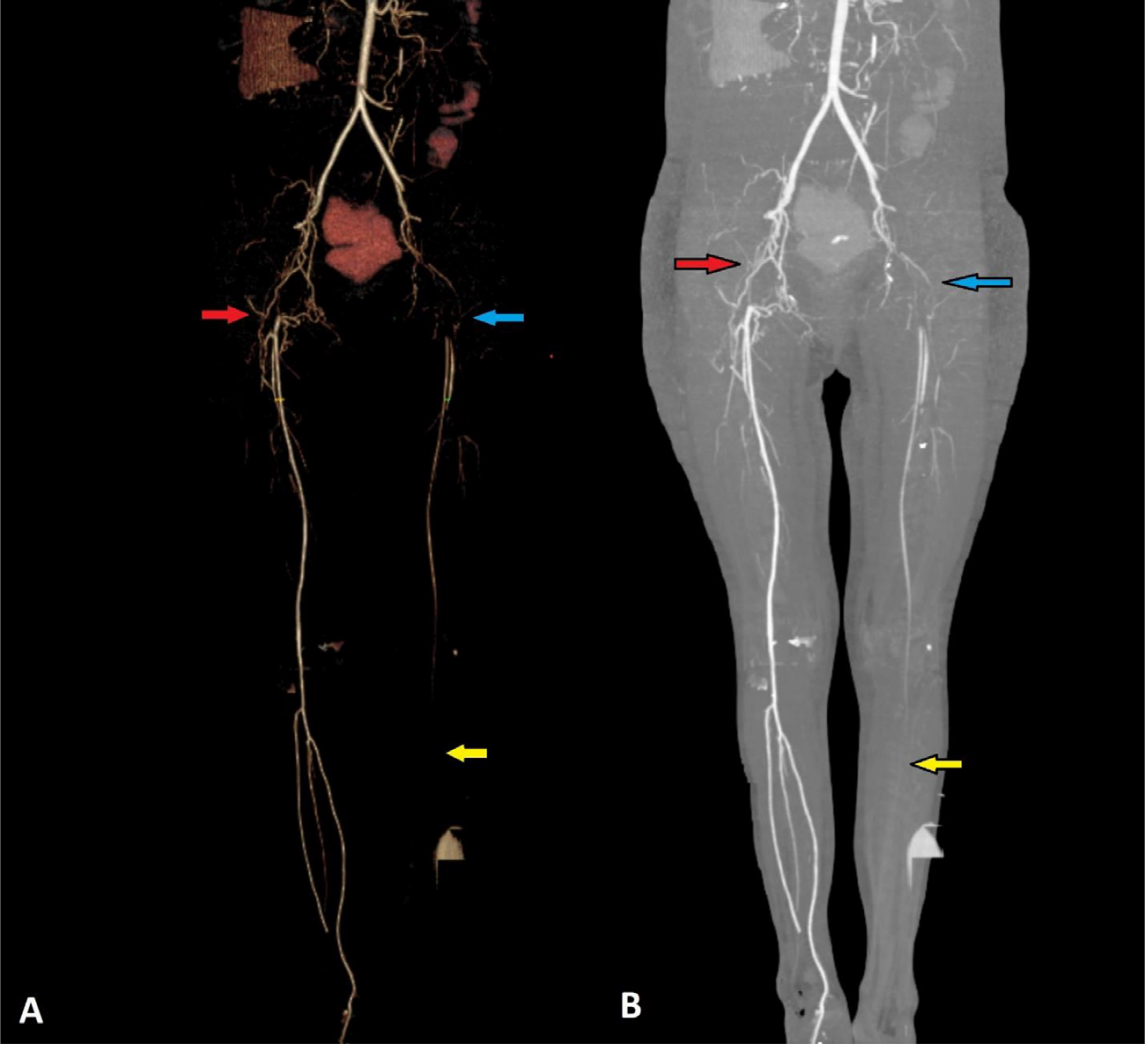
Lower limbs CT angiography, coronal view (A and B): Agenesis of the right external iliac artery led to opacification of multiple right-sided collateral vessels in the right internal iliac. (Red arrows) Complete thrombosis of the left external iliac artery (EIA) and common femoral artery (CFA). (Blue arrows) The superficial femoral artery (SFA) has decreased diameter without an obvious filling defect. The proximal parts of the anterior tibialis, peroneal, and posterior tibialis arteries have a filiform enhancement and are not opacified in other parts (Yellow arrows).
